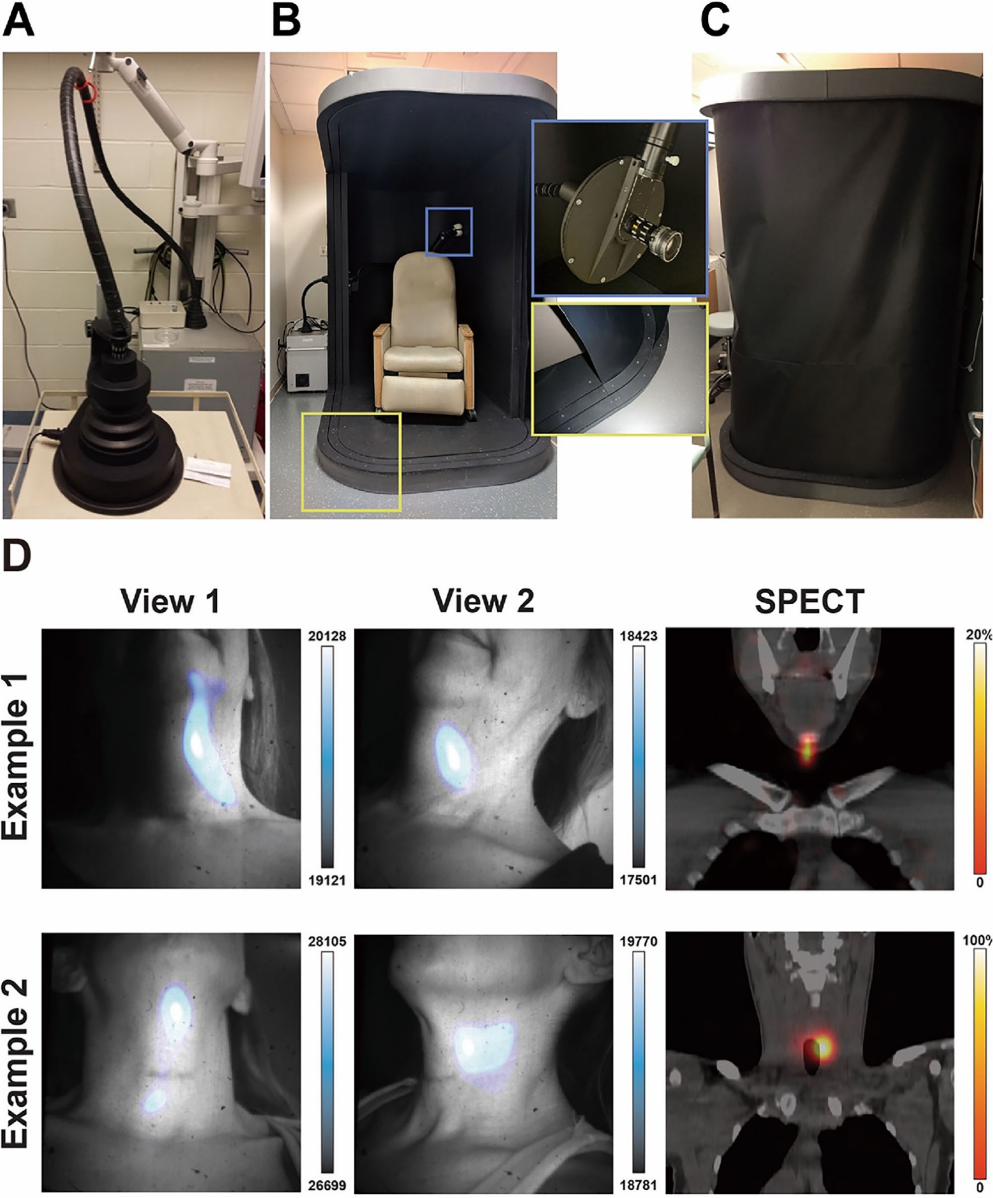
Clinical Cerenkov imaging system based on the Lightpoint fiberscope design and its application in the detection of ^131^I‐iodine in patients. A) The Cerenkov camera enclosed in a lead shielded box with a fiberscope connected to a preclinical enclosure. B) Clinical enclosure containing a reclining chair for patient imaging. The fiberscope is installed through the rear of the enclosure opposite the dual‐track shroud system. C) Both shrouds are deployed to prevent ambient light from reaching the patient during CLI. D) Example 1 and 2 display images from two angles depicting the CLI focal intensity, along with the corresponding single‐photon emission computed tomography (SPECT) images indicating uptake near the submental triangle. Reproduced with permission. Copyright 2022, Springer Nature.^[^
